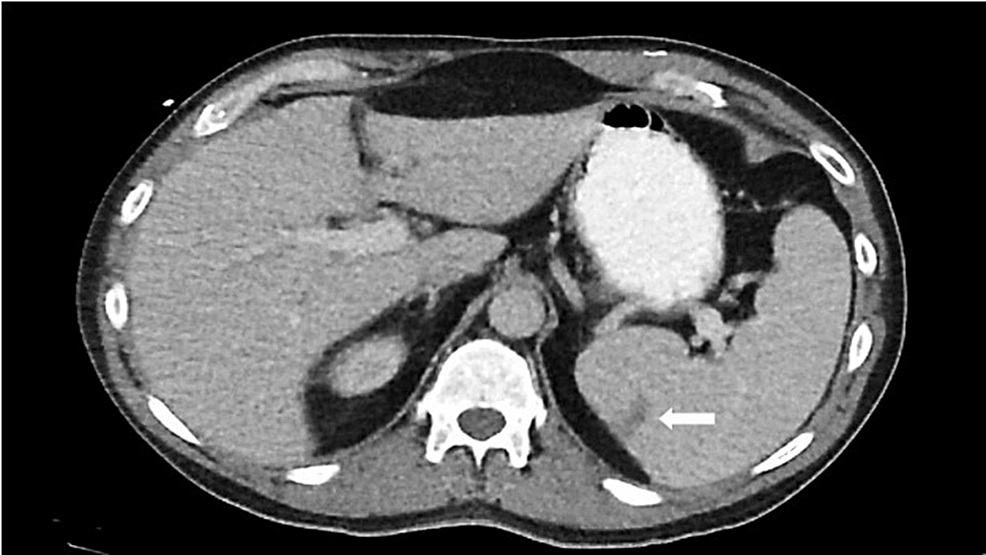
CT abdomen with contrast showing splenic infarct (arrow).
